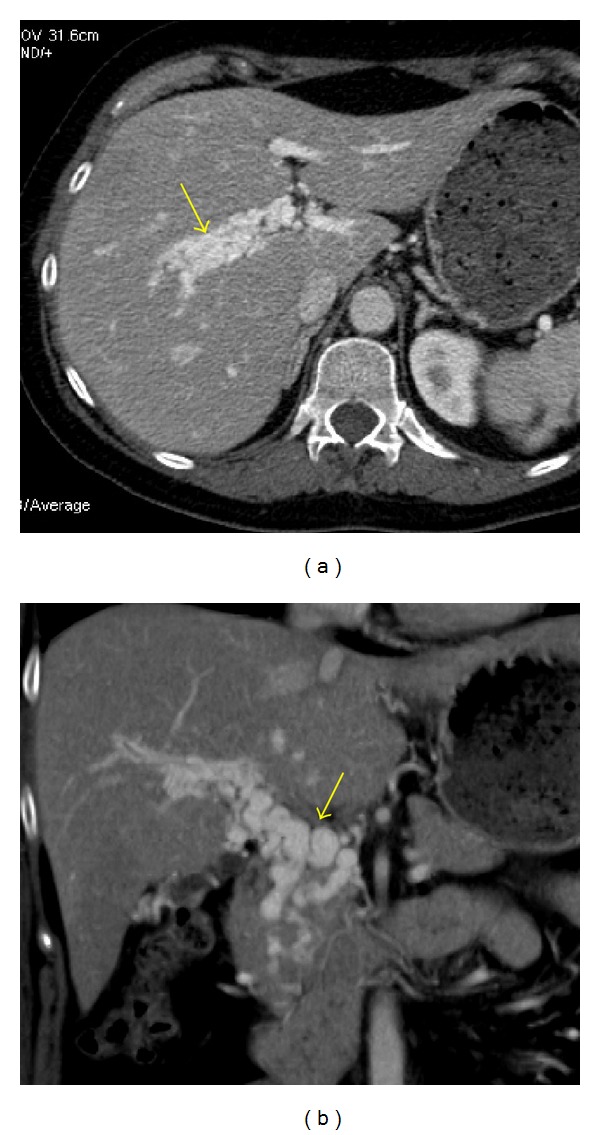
Chronic portal venous thrombosis with cavernous transformation of the portal vein. Axial (a) and coronal (b) contrast-enhanced CT images demonstrate multifocal serpiginous tubular structures in the hepatic hilum consistent with portoportal collateral vessels (arrows).
